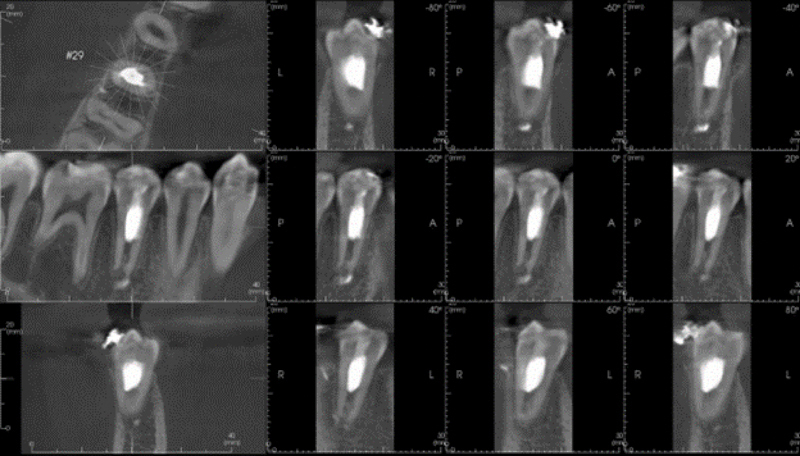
Multiple sagittal and coronal slices of cone beam computed tomography of tooth #29 displaying root endoclosure and no periapical pathology.
